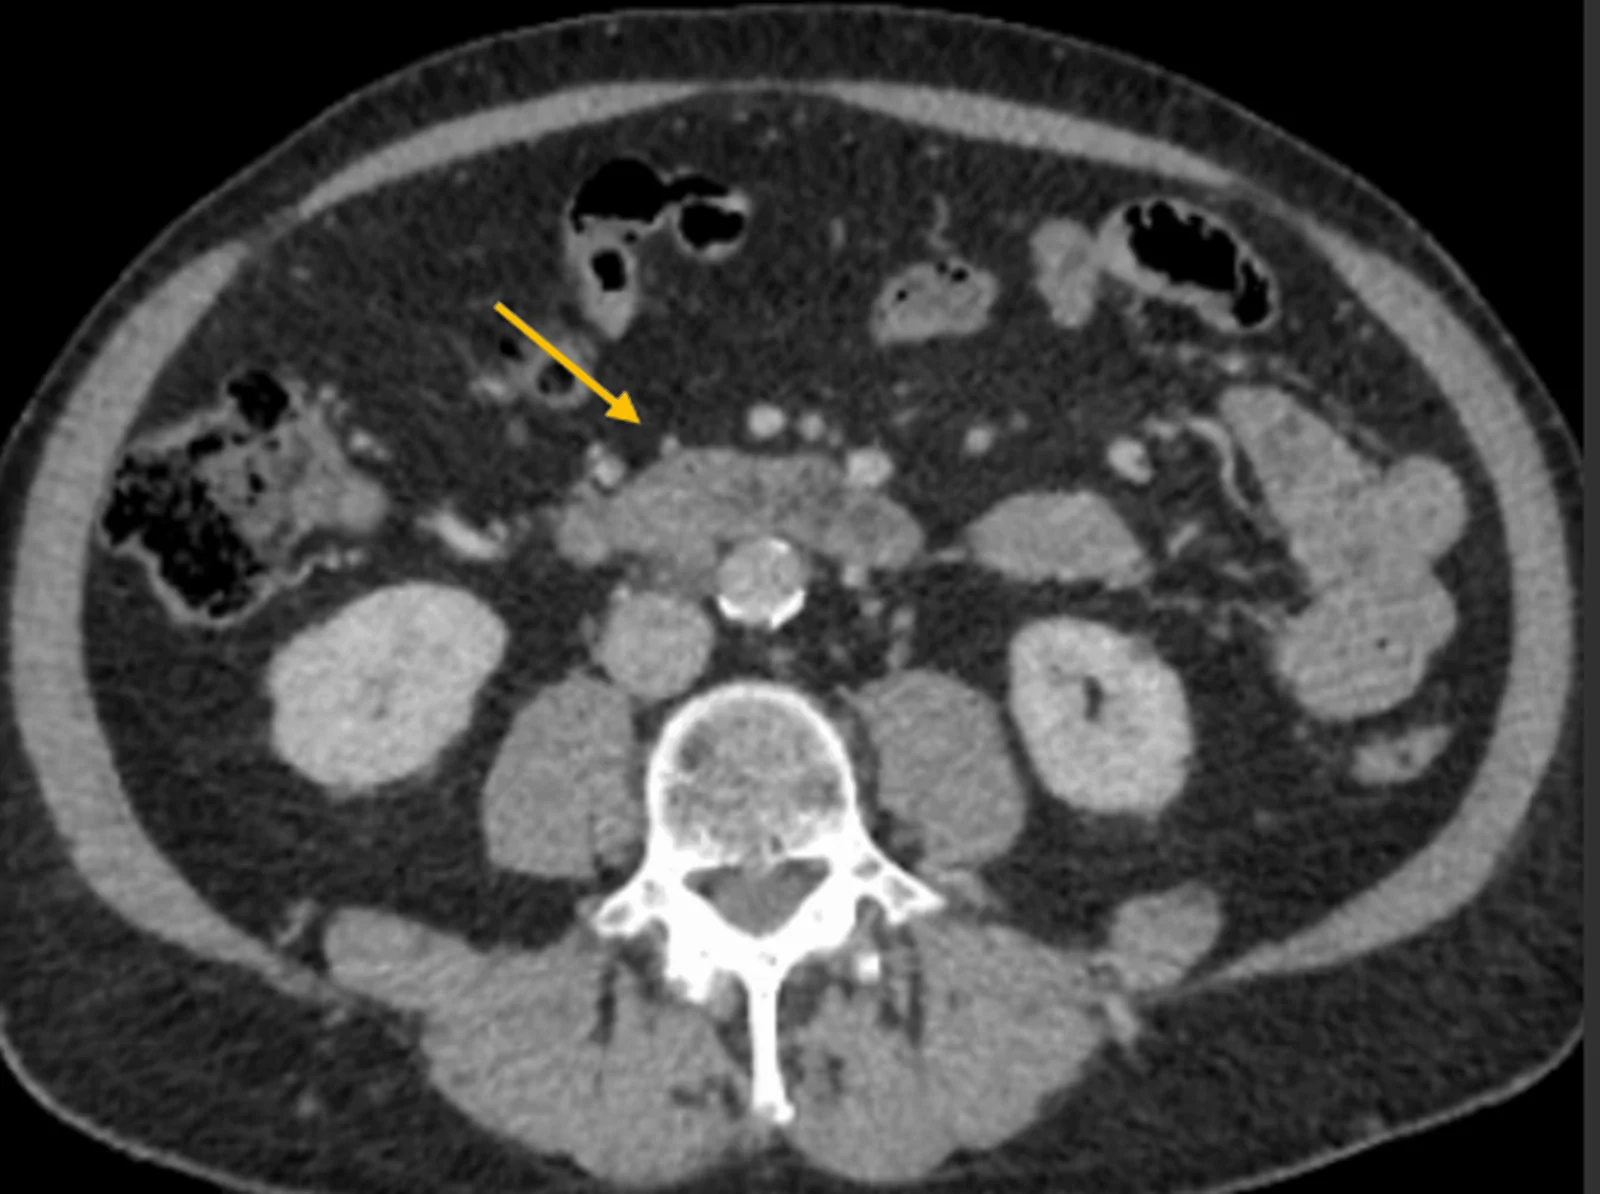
Contrast-enhanced axial CT image of the abdomen showing enlarged aortocaval lymphadenopathy (yellow arrow), considered consistent with metastatic disease following multidisciplinary team review.
